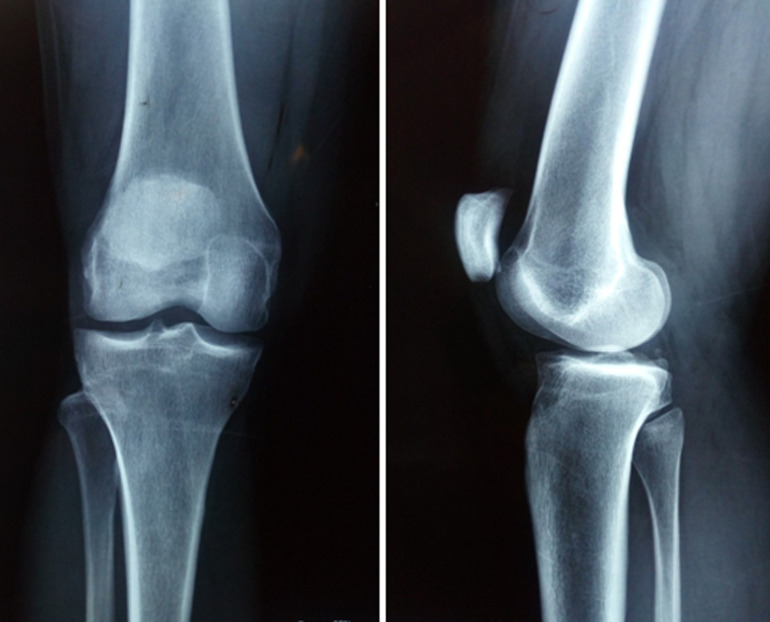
antero-posterior and lateral radiographs of the knee joint revealed no bony abnormalities
